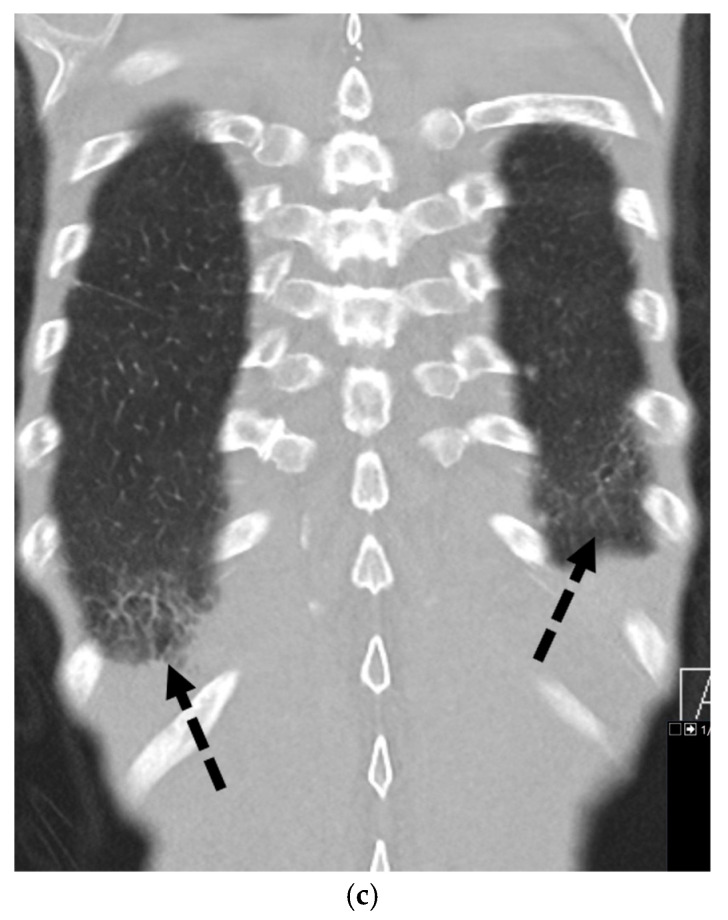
61-year-old female with systemic sclerosis. Frontal chest radiograph (a) shows a small left apical pneumothorax (open white arrow). Axial CT image in lung window (b) demonstrates diffusely dilated esophagus (white star) and small left pneumothorax (curved white arrow). Coronal CT image in lung window (c) shows basilar predominant peripheral reticulation with mild ground-glass opacity and bronchiolectasis without honeycombing (dashed black arrows), likely reflecting non-specific interstitial pneumonia (NSIP) in the setting of systemic sclerosis.
